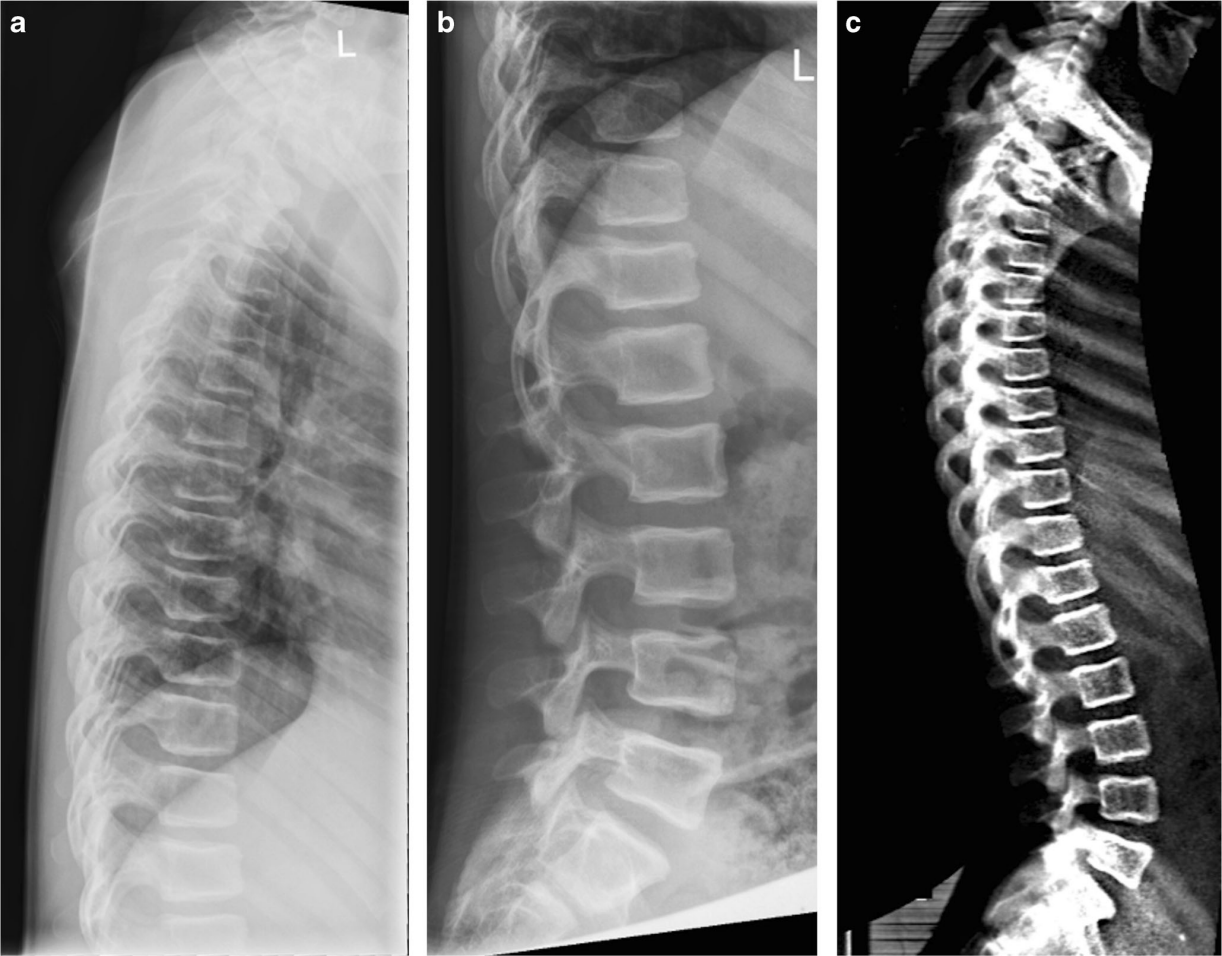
An 11-year-old boy with osteogenesis imperfecta. a-c Lateral thoracic (a) and lumbar spine (b) radiographs are juxtaposed to a lateral spine dual energy x-ray absorptiometry scan (c) performed on the same day. The image quality of (c) is non-inferior to (a) and (b), with the advantage of being a single image
